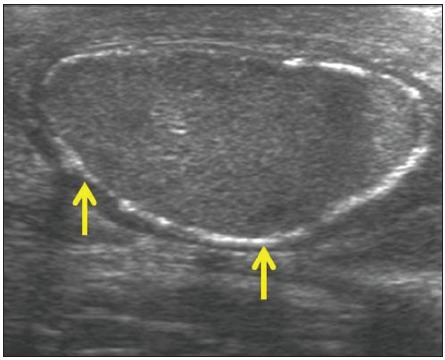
A 42-year-old man with healed testicular tuberculosis: Gray scale sonographic image demonstrates smooth peripheral calcification along the tunica vaginalis (yellow arrows). The patient had a remote history of pulmonary tuberculosis, which was treated with antitubercular drugs.
